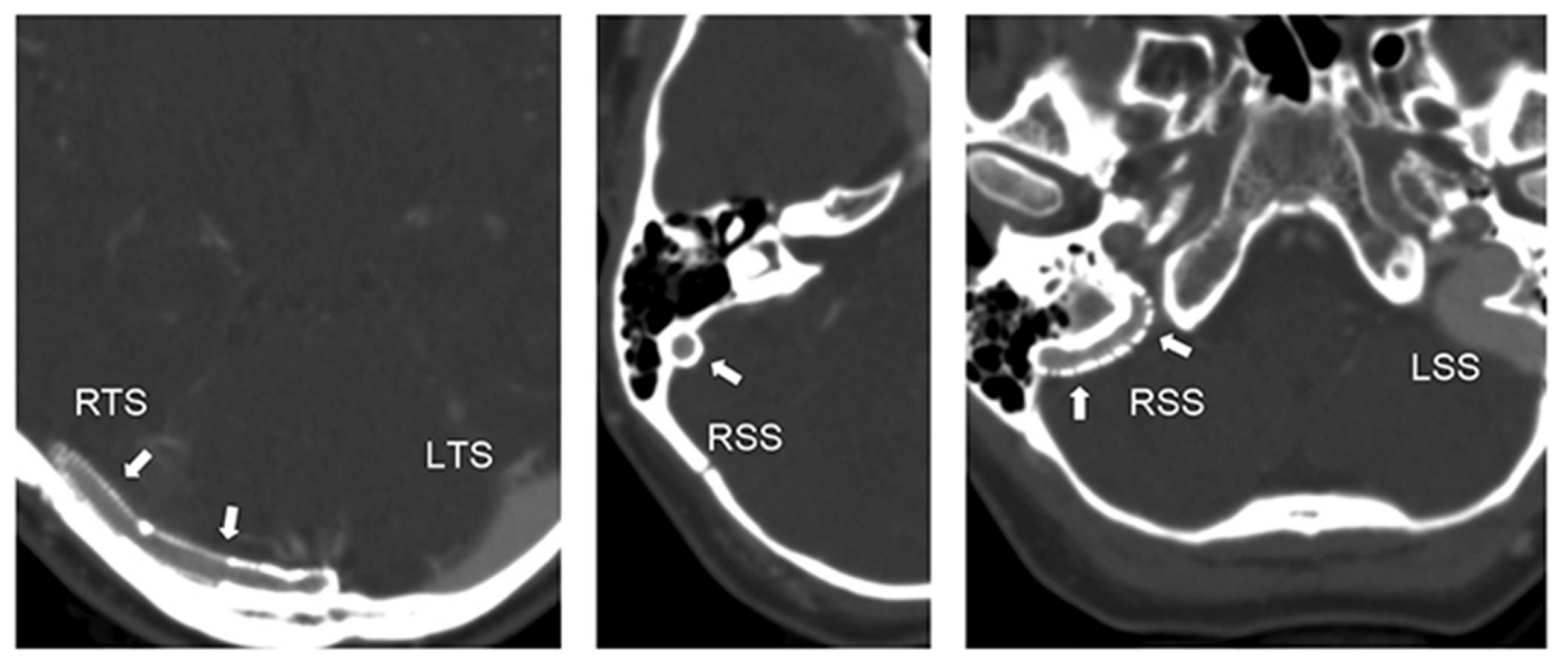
Follow-up CT venogram of the posterior fossa Axial scans at different levels showing contrast opacification inside the stents (arrows). Left-hand image shows stents in the right transverse sinus (RTS), middle image in the right sigmoid sinus (RSS) and right-hand image at right sigmoid sinus outflow into the jugular vein. Note the normal transverse and sigmoid sinuses sinuses on the left side (LTS, LSS).
